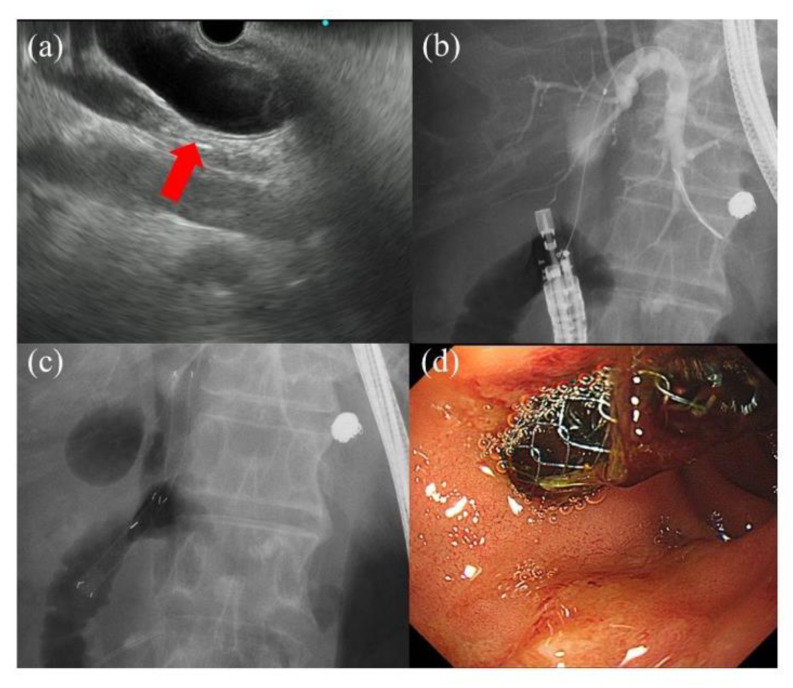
A case of endoscopic ultrasound-guided biliary drainage for a patient with distal malignant biliary obstruction. (a) Endoscopic ultrasound shows the dilation of the common bile duct (red arrow) (CBD). (b) After puncturing the CBD, successful cholangiography is performed. (c,d) A braided-covered self-expandable metal stent (10 mm diameter, 8 cm length; WallFlex biliary RX fully covered stent; Boston Scientific, Natick, MA, USA) is placed.
